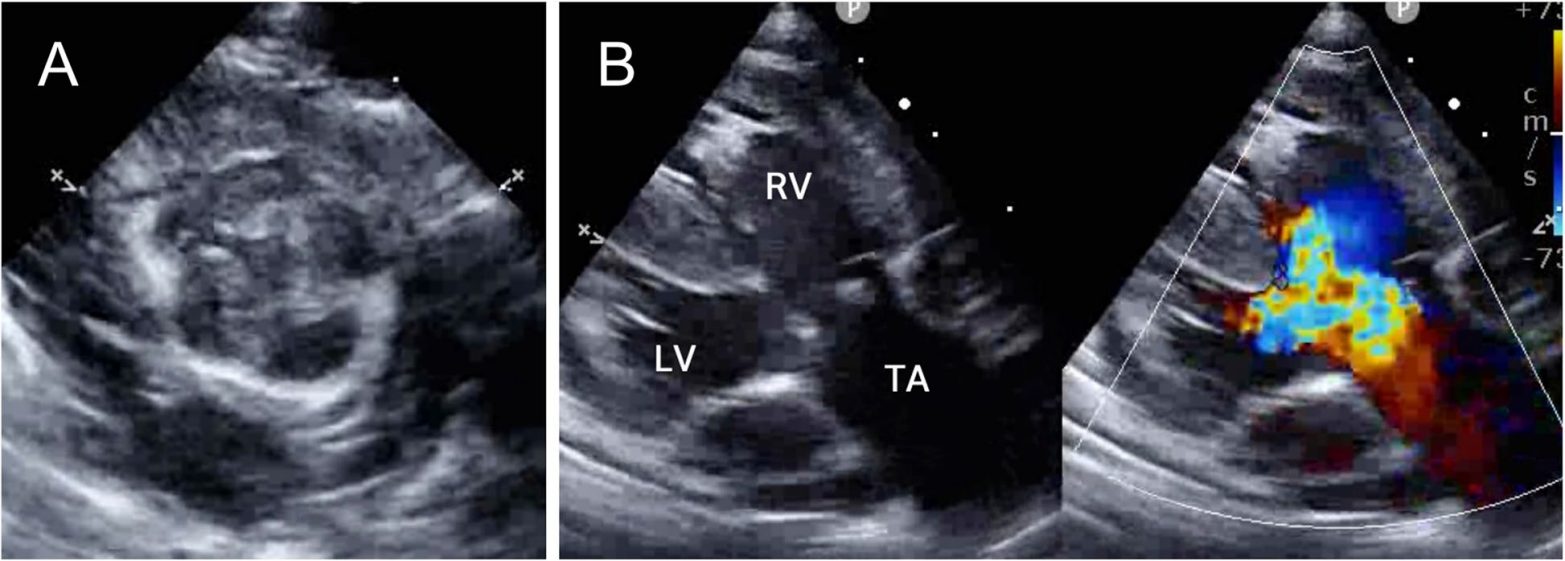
A Preoperative truncal valve in the parasternal short-axis view. B Color Doppler echocardiography shows significant truncal valve regurgitation. RV, right ventricle; LV, left ventricle; TA, truncus arteriosus
